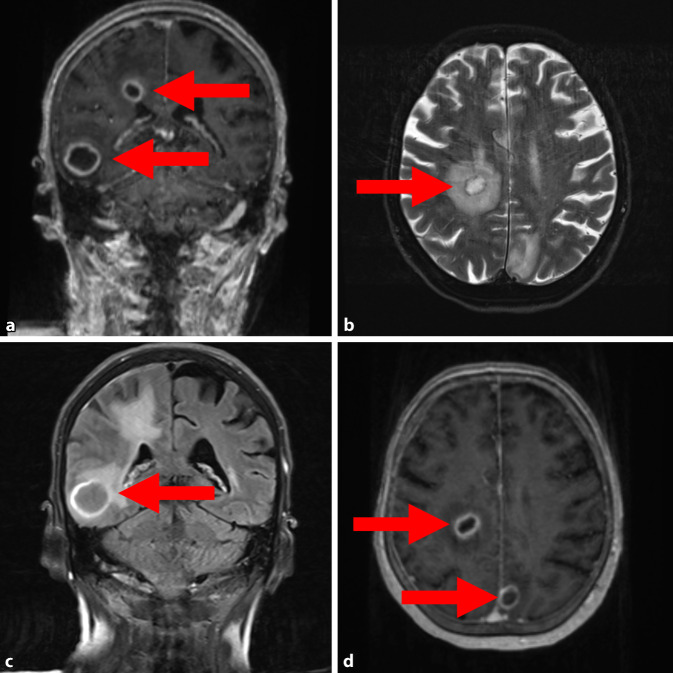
Multiple intrakranielle Abszesse (*Pfeile*) in der cMRT, die durch paradox-septische Embolien entstanden sind, jeweils markiert durch *Pfeile*. a Post-KM MP-RAGE koronar. b T2 TSE FS axial. c FLAIR koronar. d Post-KM MP-RAGE axial
